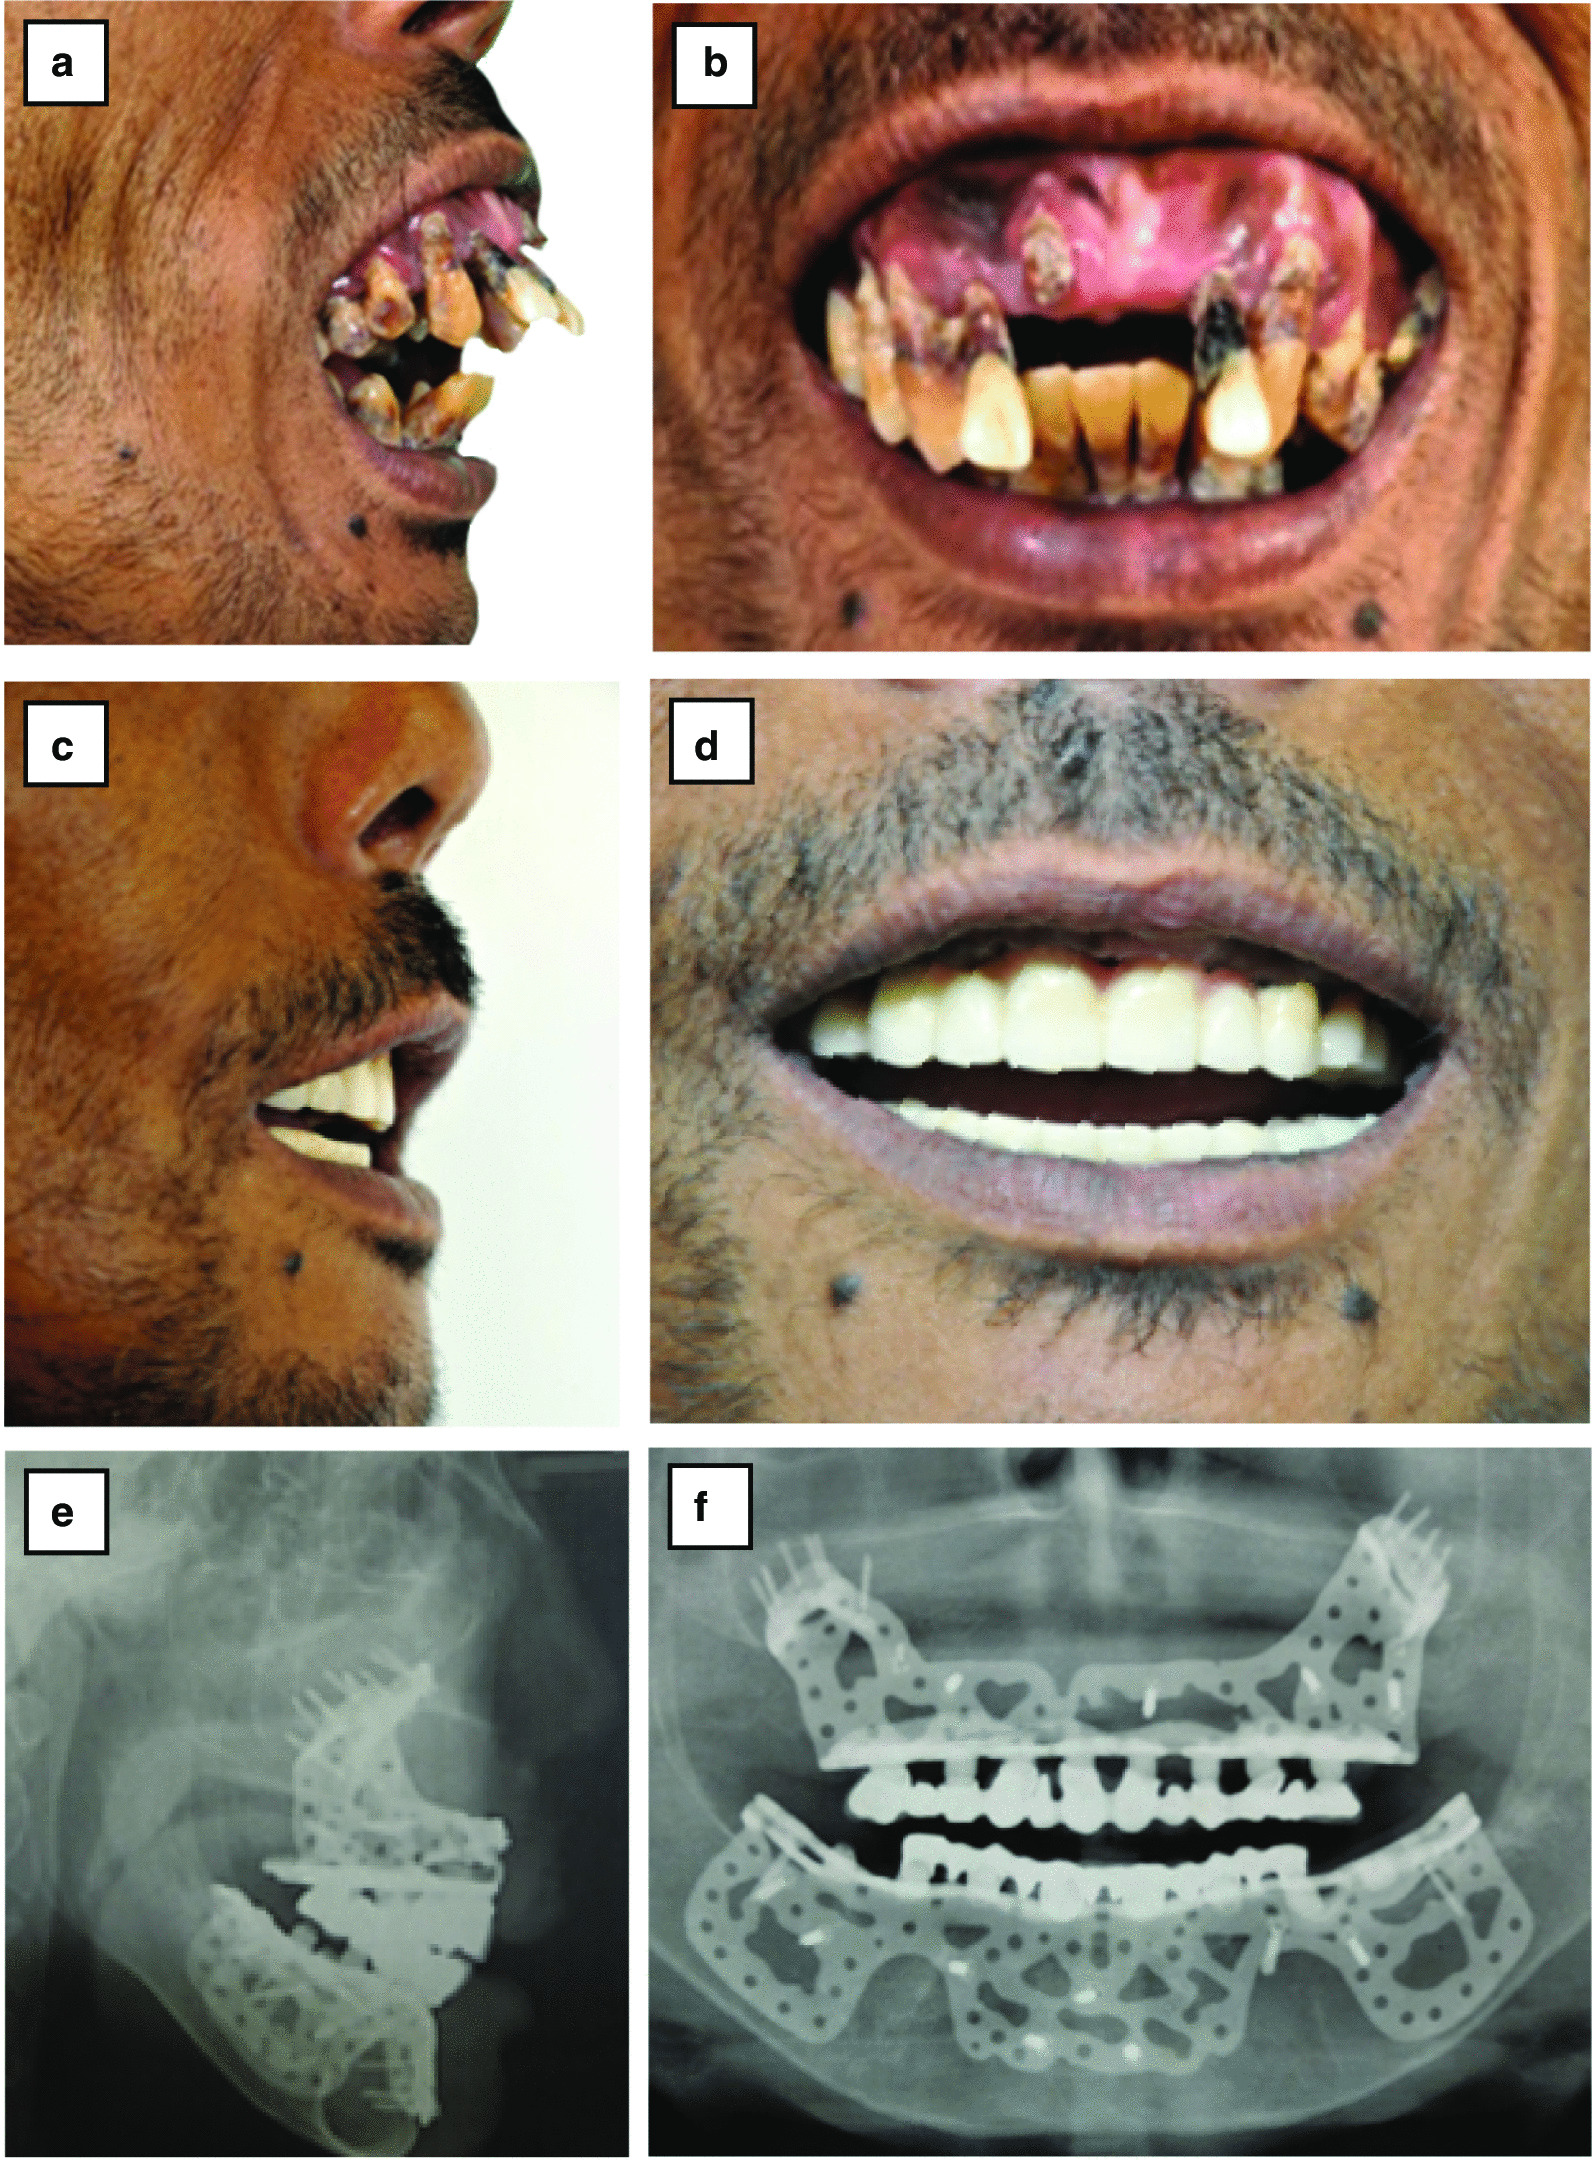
Comparison of preoperative a and b and postoperative lateral and frontal view. c and d Post-rehabilitation picture of upper and lower jaw fixed prosthesis. e and f Postoperative radiographic X-ray. e and f Panoramic lateral cephalometric view X-rays
